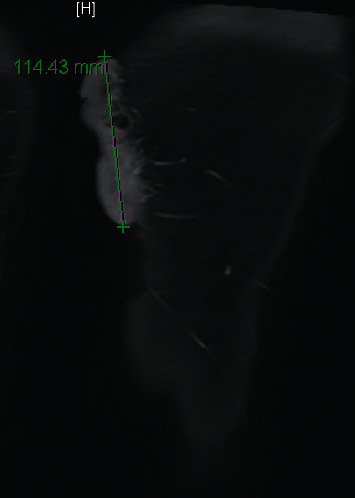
MRI imaging of the left thigh showing posteromedial mass, with arterial blood supply from branches of the deep femoral artery.
